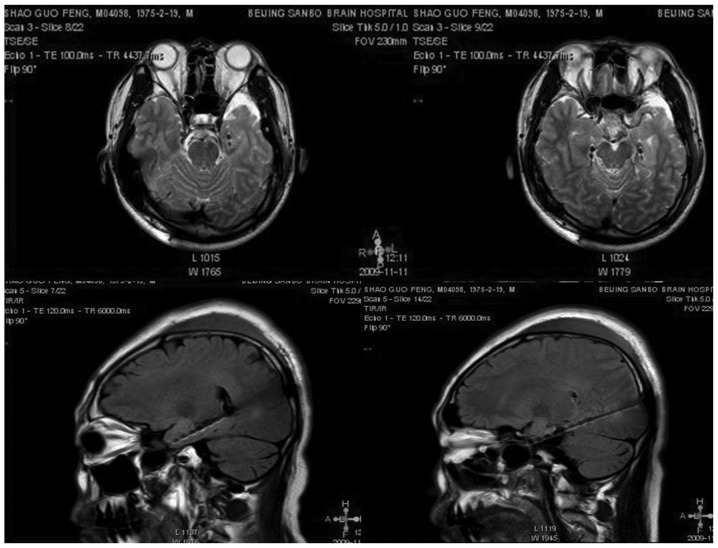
Post-surgery magnetic resonance imaging.
